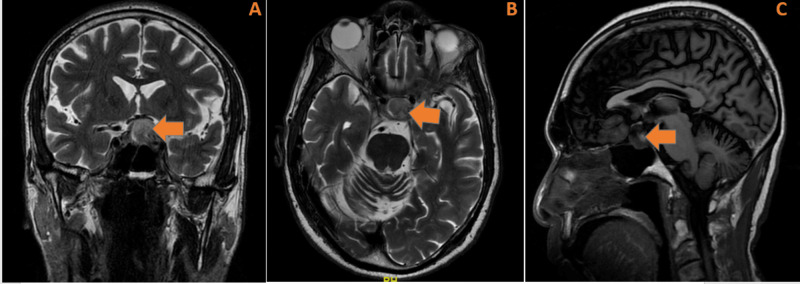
MRI images in different views. A) T1-weighted-fluid-attenuated inversion recovery MRI (coronal view), B) T2-weighted-FSE (axial view), C) T1-weighted-fluid-attenuated inversion recovery (sagittal view), showing heterogeneously enhancing mass within the sella turcica measuring 1.6 x 1.9 x 1.7 cm as shown by orange arrows causing elevation of the optic chiasma and laterally extending into the cavernous sinus. FSE: Fast Spin Echo
